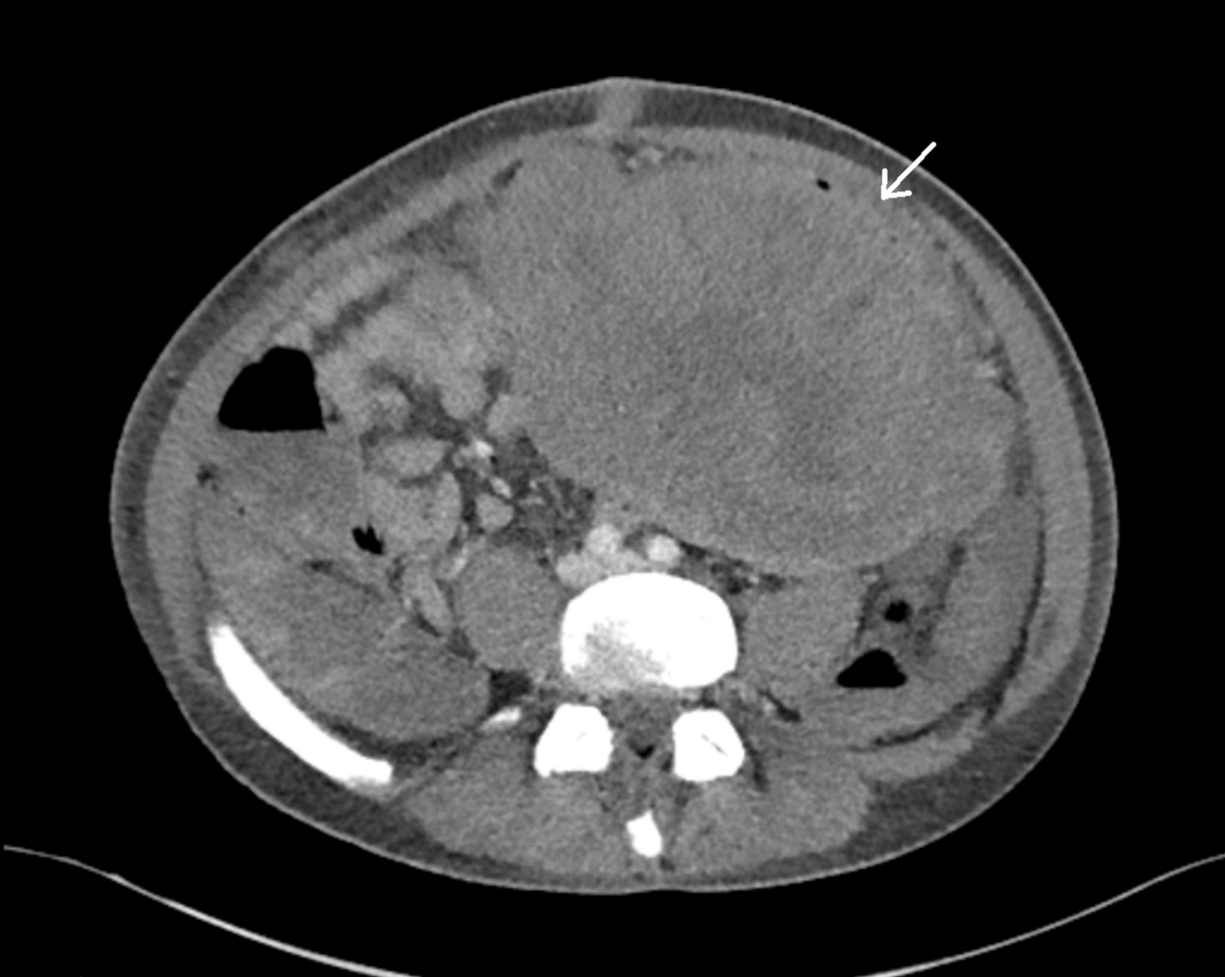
Axial CT scan of the MRT White arrow indicating the intra-abdominal tumor CT, computed tomography; MRT, malignant rhabdoid tumor
